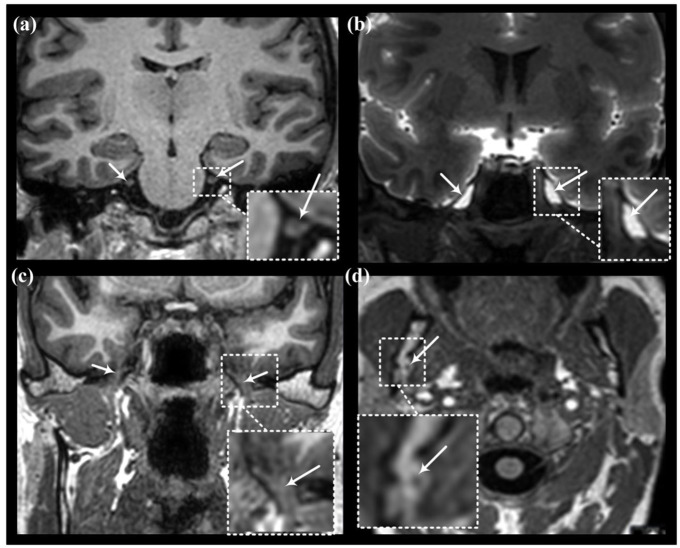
Slice sections and measurement areas along the trigeminal nerve utilizing T1-weighed MRI. Images demonstrate slice sections of the trigeminal nerve and branches at (a) prepontine subarachnoid space in coronal plane, (b) Meckel’s cave in coronal plane, (c) foramen ovale in coronal plane, and (d) mandibular bone in axial plane. White arrows point at the nerve segments; for Meckel’s cave the region of interest was within the cave itself. MRI: magnetic resonace imaging.
